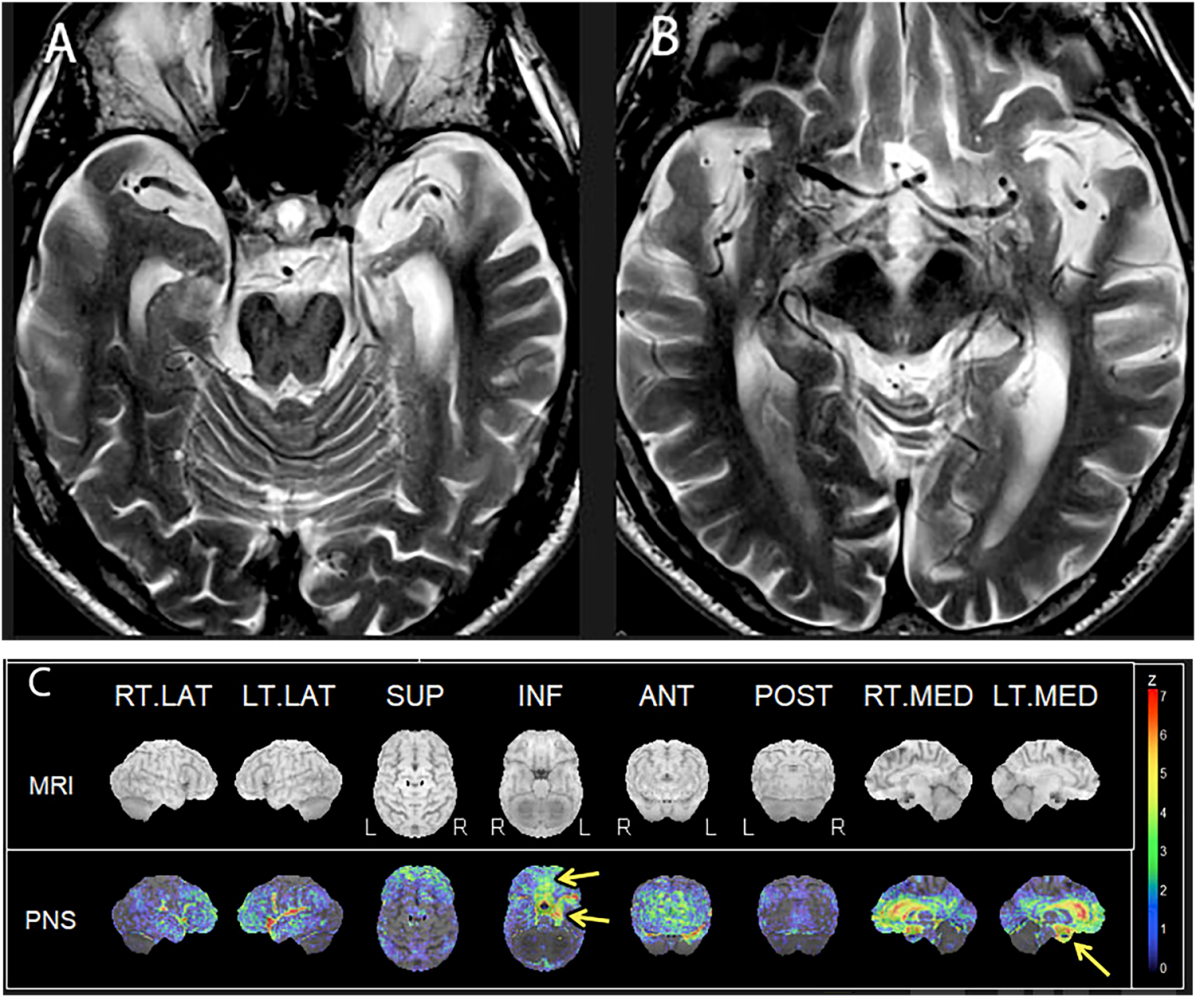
83-year-old male depressed since COVID, with a history of hypertension, hyperlipidemia, memory loss, word-finding difficulty, but still uses his cell phone. T2-weighted axial images of the hippocampus (A) and inferior temporal lobe (B) show severe atrophy of the hippocampus and amygdala, left greater than right. Furthermore, there are prominent sulci over the orbitofrontal cortex bilaterally. FDG-PET (C) with 3D-SSP reconstruction shows severe hypometabolism in the hippocampus and medial temporal lobes, left greater than right, and metabolic reduction in the medial orbitofrontal gyri (yellow arrows). The findings are consistent with LATE (limbic-predominant age-related TDP-43 encephalopathy)
